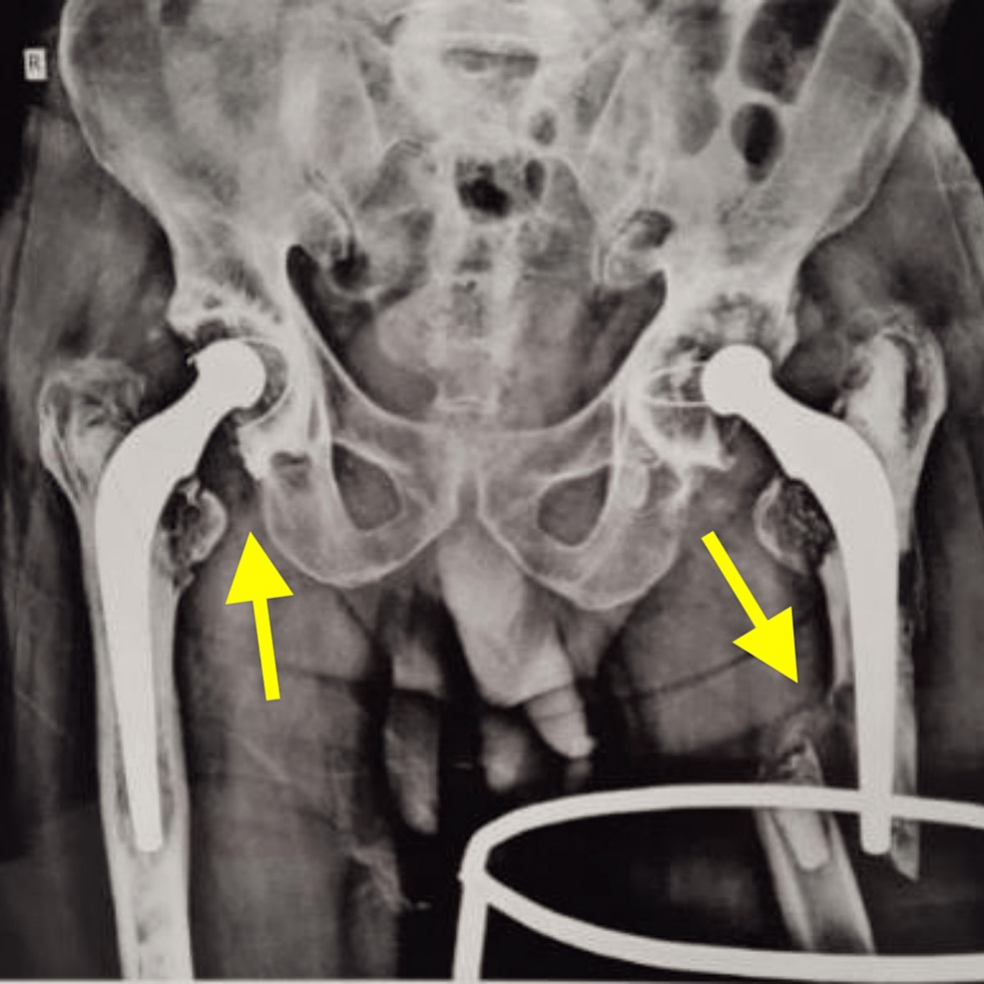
Pre-operative radiograph of pelvis with both hip joints (PBH) anteroposterior view shows bilateral total hip arthroplasty with periprosthetic femur fracture left side
